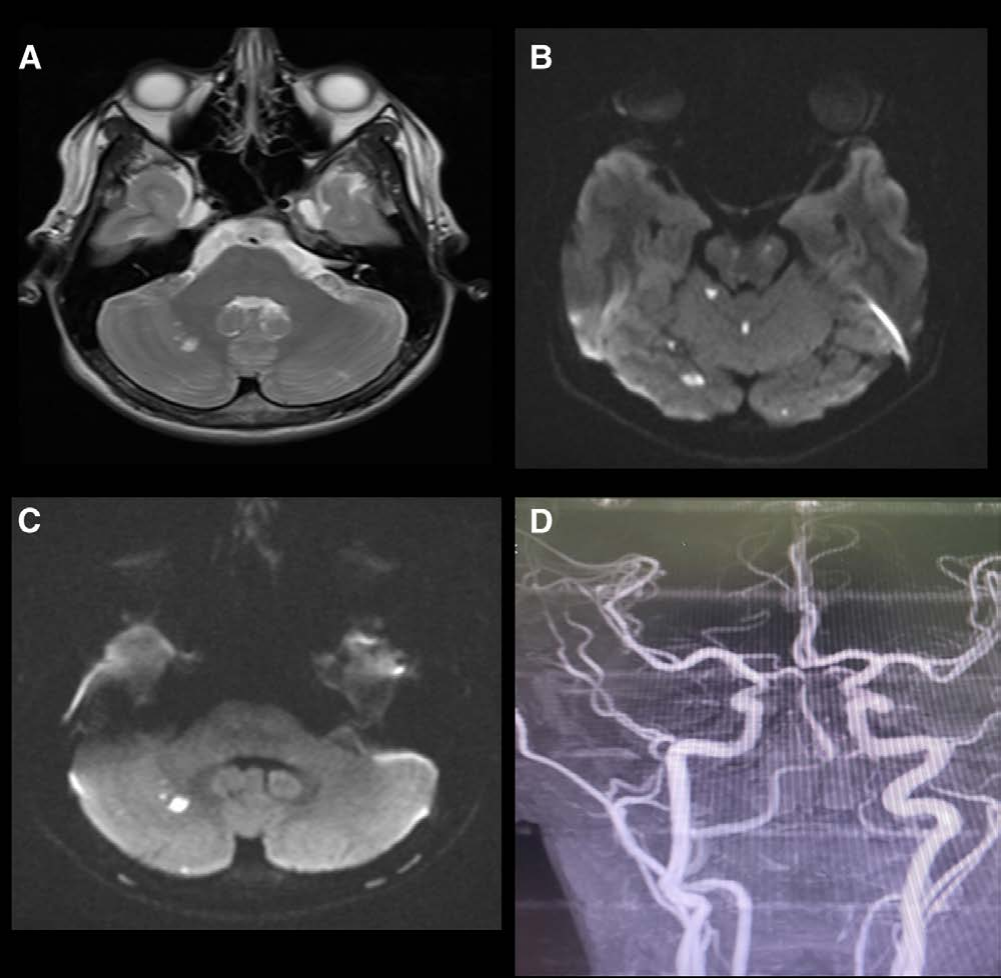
Brain MRI posterior circulation multiple embolic infarcts: (A and B) diffusion weighted images at different levels, (C) T2-weighted image show abnormal T2 discrete hyperintensity foci with diffusion restrictions involving the cerebellum and occipital lobes bilaterally, (D) left vertebral artery narrowing.
